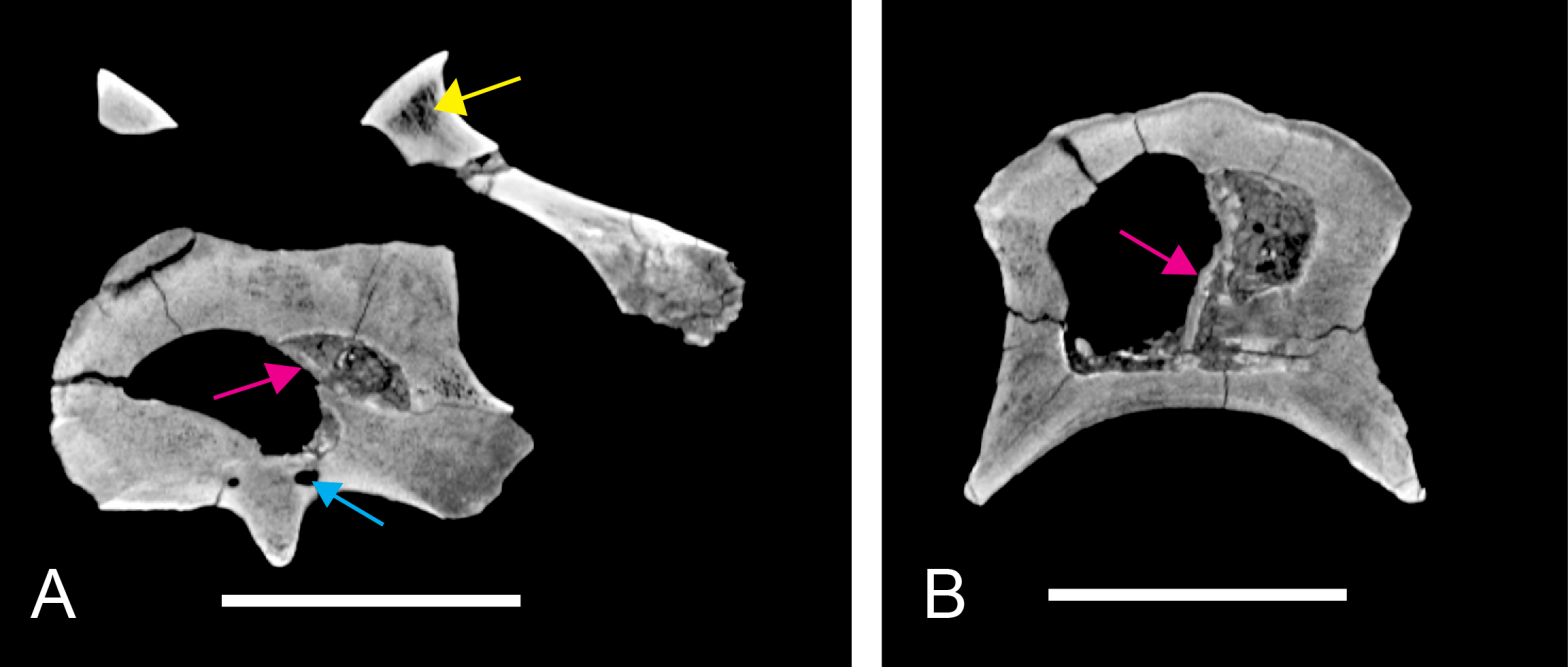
CT images of BSPG 2006 I 54. (A) slice through anterior part of the vertebra in popsterior view. (B) slice through centrum in dorsal view. Pink arrows indicate course of bony septum separating two large camerate chambers. The yellow arrow indicates hollow structure in prezygapophysis. The blue arrow indicates accessory foramen underneath transverse ridge. Scale bars equal 10 cm.
